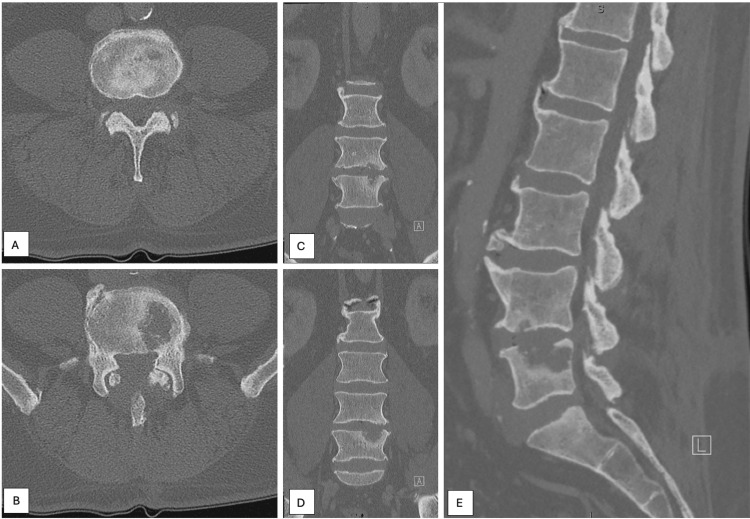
Repeat non-contrast computed tomography of the lumbar spine was performed 47 months after cancer diagnosis and demonstrated a marked interval expansion of the erosive L5 lesion with poorly defined margins, which now measured 30.4×22.6×13.8 mm. In addition, a hypodense, erosive lesion in the left side of L4 was also identified, which measured 4×13.9×4.3 mm. Figure 6A demonstrates an axial cut at the level of the L4 vertebral body lesion, while Figure 6B demonstrates the lesion in the L5 vertebral body. Figures 6C and 6D demonstrate coronal cuts, with Figure 6C showing both lesions and demonstrating a more anterior cut than the image presented in Figure 6D. Figure 6E demonstrates the L4 and L5 vertebral body lesions on a sagittal image.
